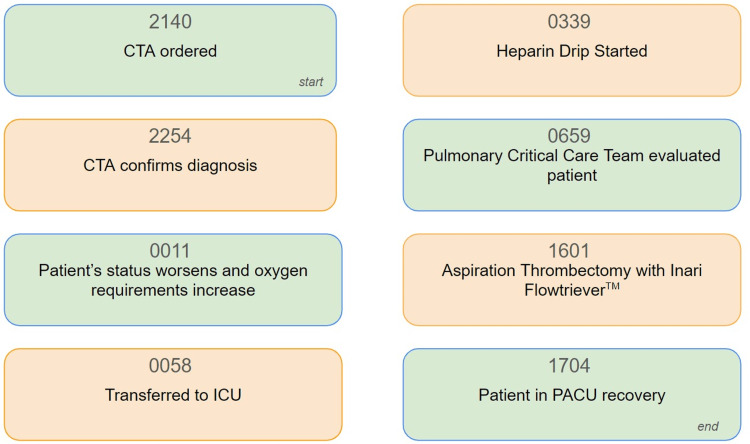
Timeline of events from ordering confirmatory imaging to completion of procedure CTA: computed tomography angiography; ICU: intensive care unit; PACU: post-anesthesia care unit
